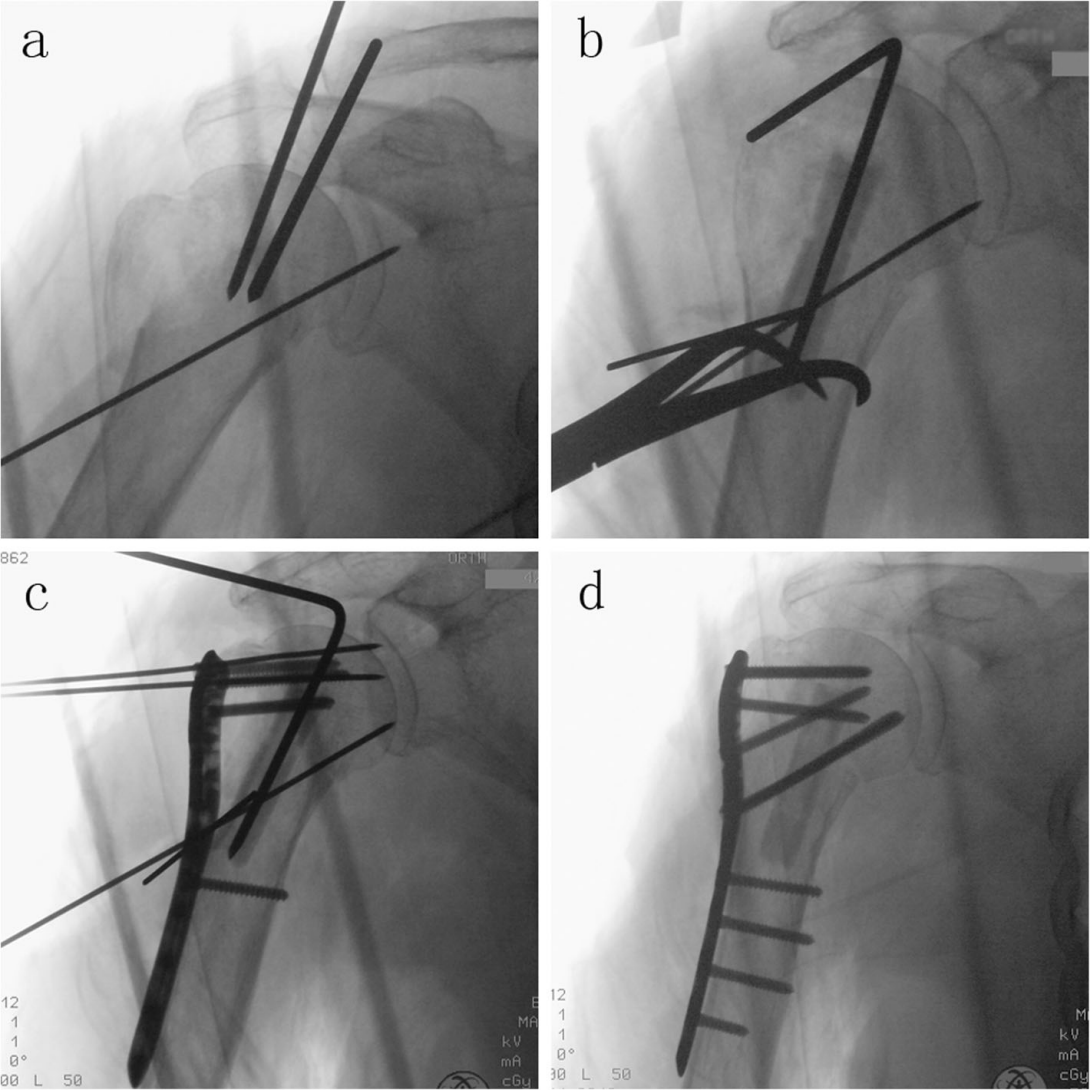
The reduction and fixation process with a fibular strut allograft. a A 1.5 mm guidewire was placed 1 cm posterior to the intertubercular groove and 1 cm medial to the transition between the head and the greater tuberosity. b The fibular allograft was inserted into the cavity, through the fracture site, through the guidewire. And then, it was pushed upwards to support the humeral head in a proper height. c After confirming the fracture reduction, a LCP was used to fix the fragments. Locking screws were placed through the graft into the humeral head and shaft. d Post-operative radiograph showing good anatomical reduction
